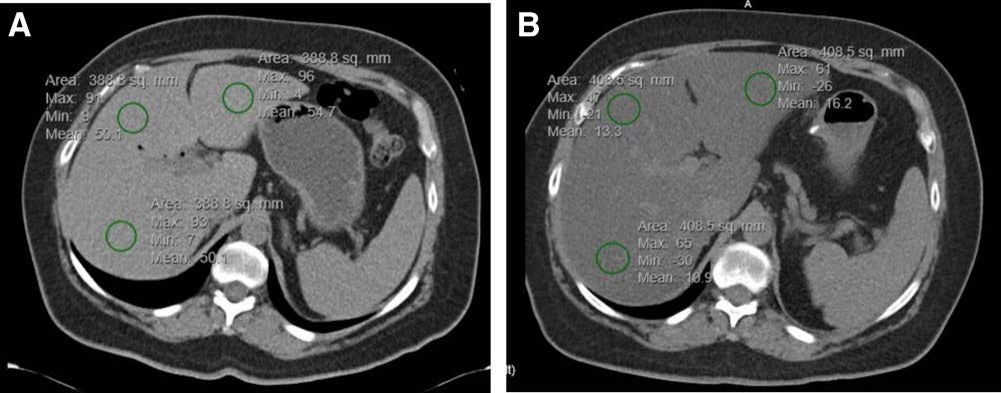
Non-contrast enhanced pre-operative (A) and post- operative (B) CT images of a representative patient with a malignant indication for surgery with interval development of hepatic steatosis in 22.3 months.
